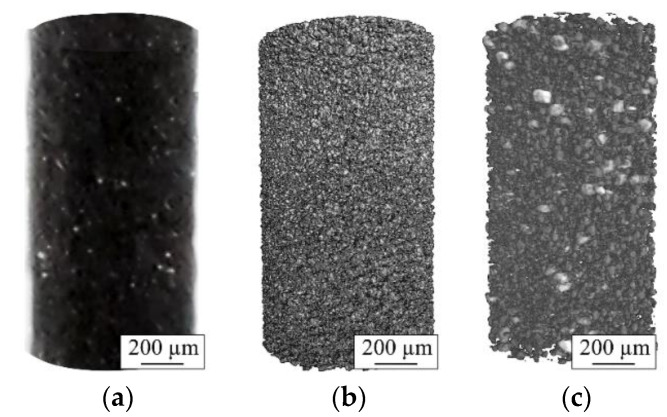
(a) Prepared cylindrical powder sample for the CT investigation and the 3D images of the scanned (b) AdPre and (c) AdEle powders.
